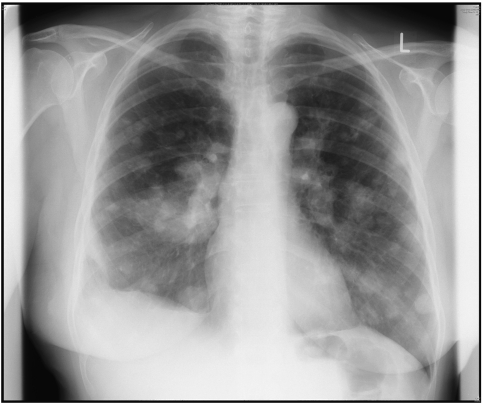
An ‘unequivocal’ true positive. Chest X-ray showing multiple metastases throughout both lungs in a patient with stage IV breast cancer.
